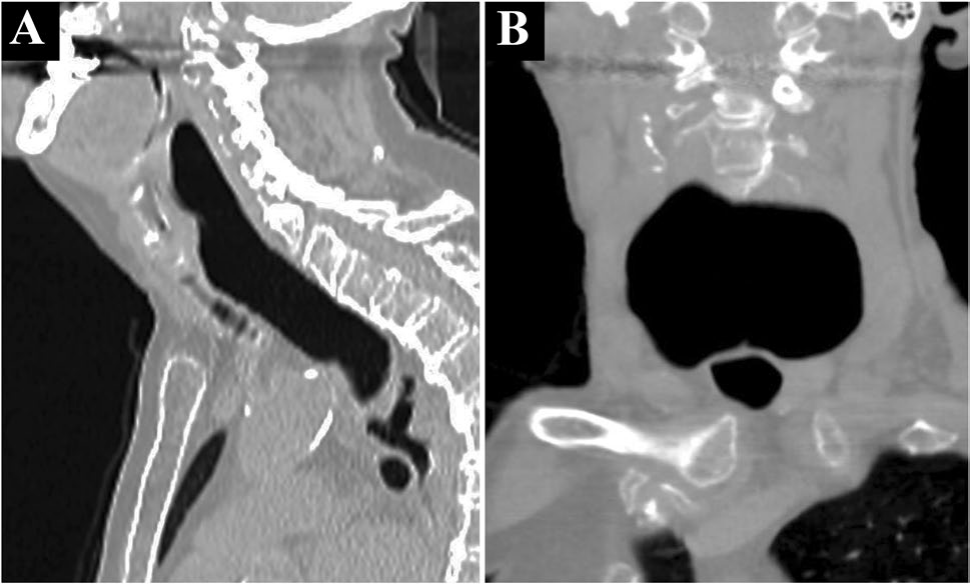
CT scan of the neck, in lateral (a) and anterior–posterior (b) direction, showing the large dilatation of the esophagus
